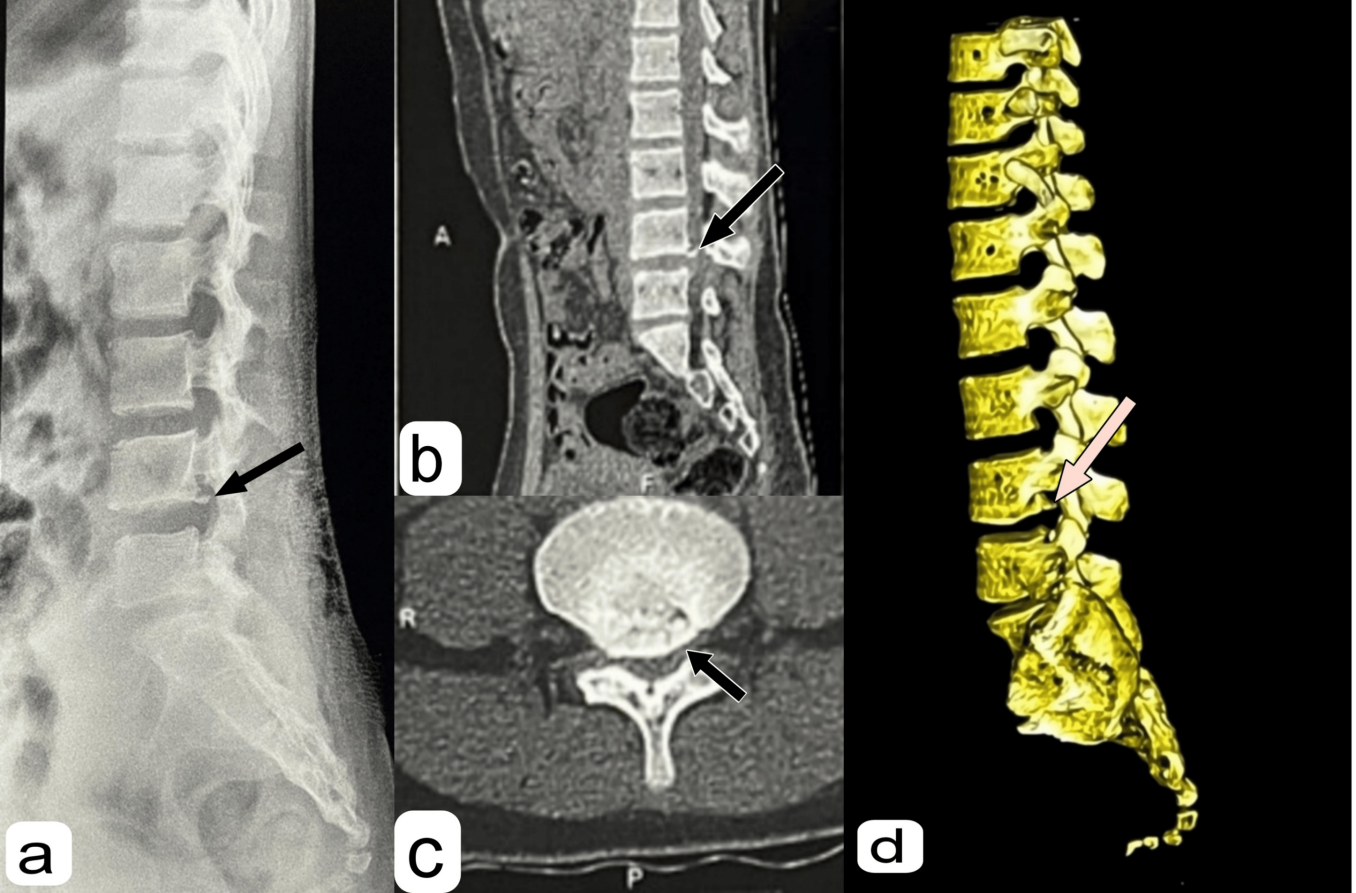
(a) X-ray of the LS spine lateral view, (b) sagittal section of CT of the LS spine, (c) axial view of L4 CT, and (d) 3D CT of the LS spine (a) X-ray of the LS spine lateral view shows a PRAF fragment at the posterior caudal end of the L4 vertebra (black arrow). (b) The sagittal section of the CT of the LS spine shows a PRAF fragment (black arrow). (c) The axial section of the CT at L4 shows the PRAF fragment (black arrow). (d) 3D CT of the LS spine showing the PRAF fragment (pink arrow). LS: lumbosacral, PRAF: posterior ring apophyseal fracture, CT: computed tomography
